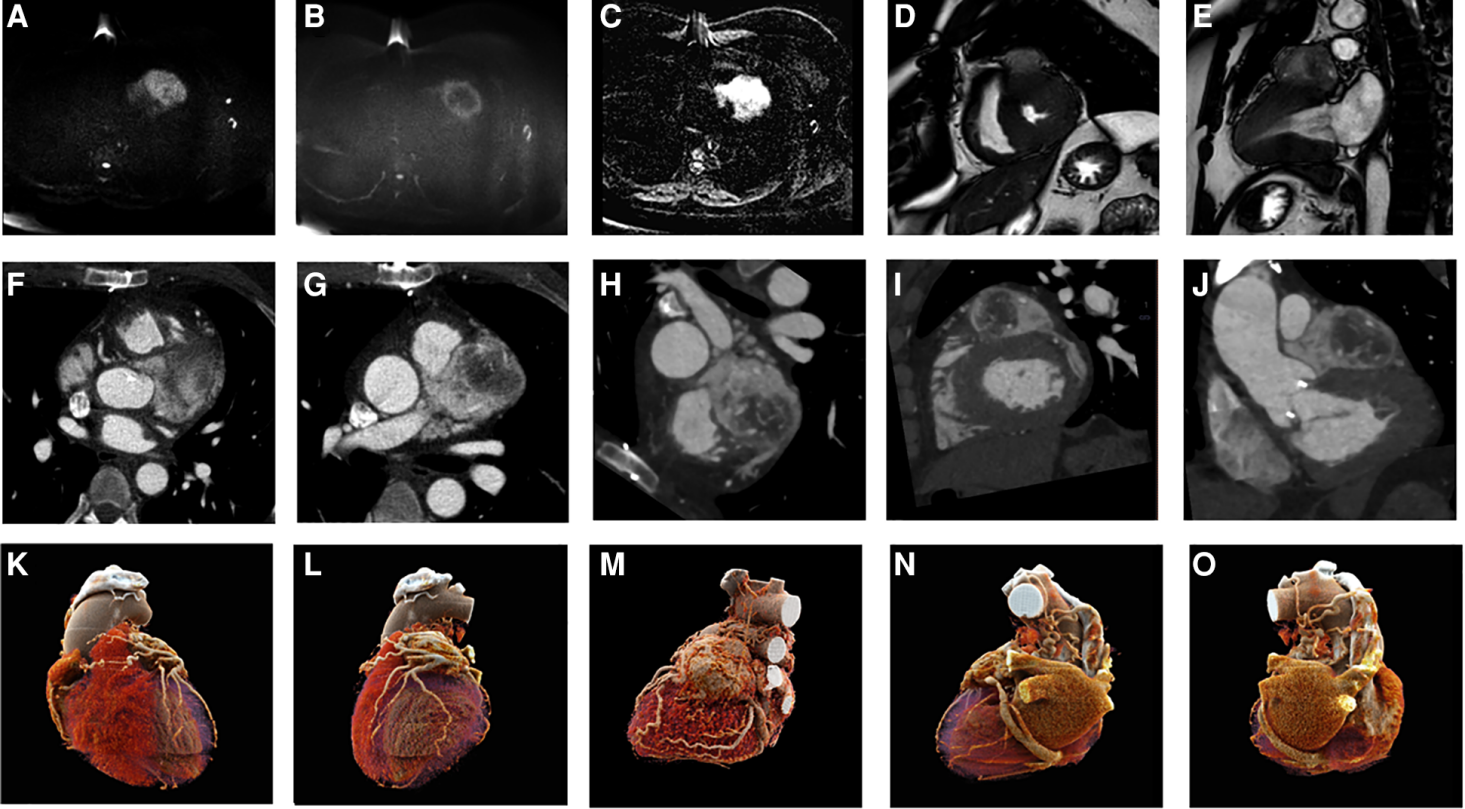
CMR acquisition (A–E): DWI b 0 and b 1,000 (A,B) and ADC map (C) showing high cellularity of the lesion; balanced gradient echo sequence in short axis and two chambers (D,E), showing morphology and extension of a PGL. CCT acquisition (F–J): axial (F,G) and oblique planes highlighting the morphology, position, and contrast of the PGL. CR reconstructions in different planes (K–O).
